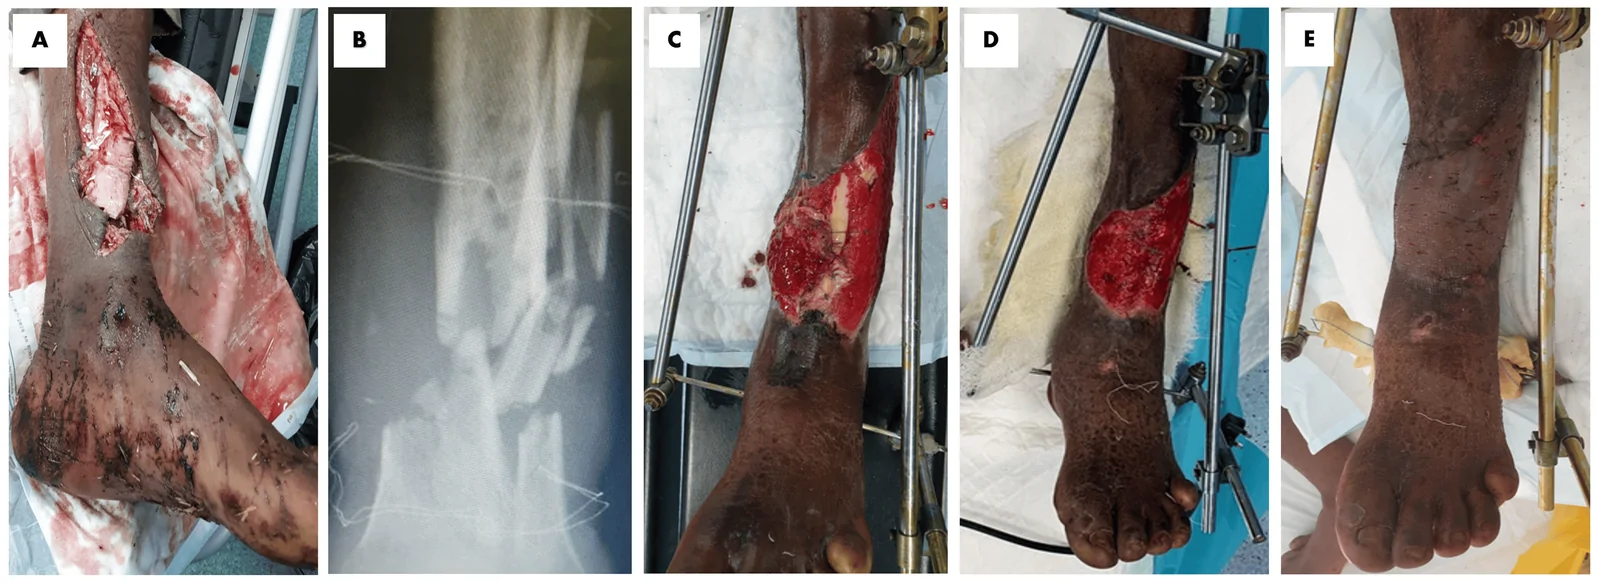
Salvage peroneus brevis flap after partial soleus flap loss. (A) Contaminated donkey-bite/crush wound at presentation. (B) Anteroposterior radiograph showing a highly comminuted displaced fracture of the distal tibia and fibula. (C) Residual distal defect with re-exposed bone after partial distal soleus flap loss. (D) Complete coverage after the inset of the adjunct distal-based peroneus brevis muscle flap alongside the surviving soleus muscle. (E) Stable coverage with a satisfactory split-thickness skin graft takes two weeks after discharge.
